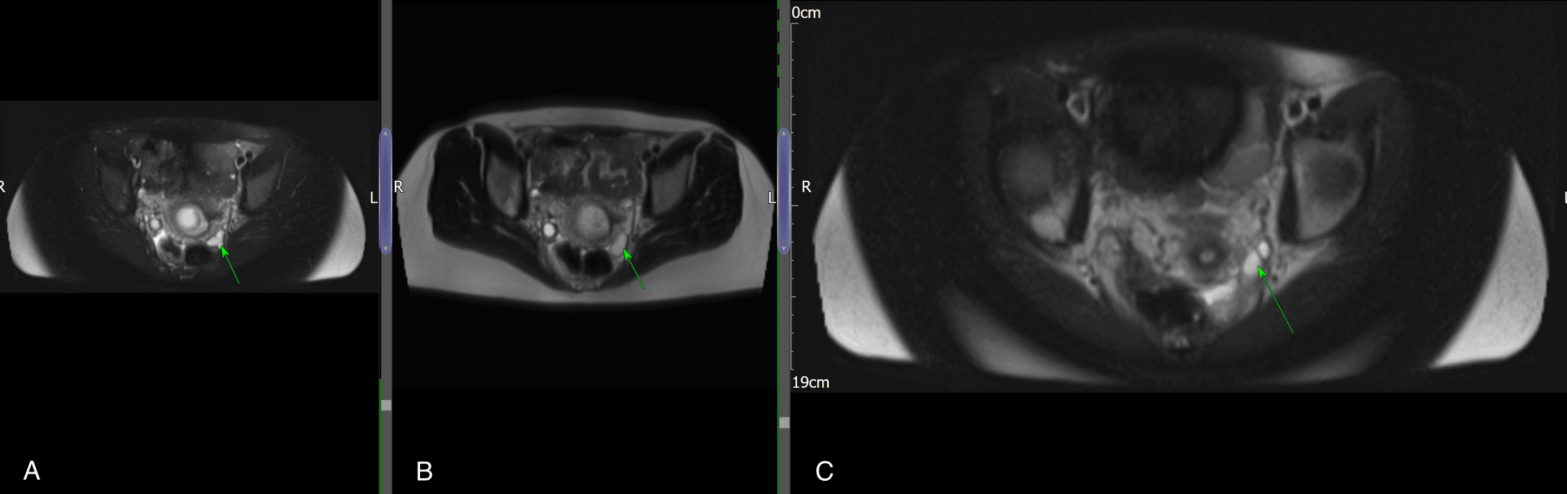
(A-C) Axial T2-weighted MRI sequences showing the sequential appearance of a tubular structure in the left adnexa, raising suspicion for a haematosalpinx.
